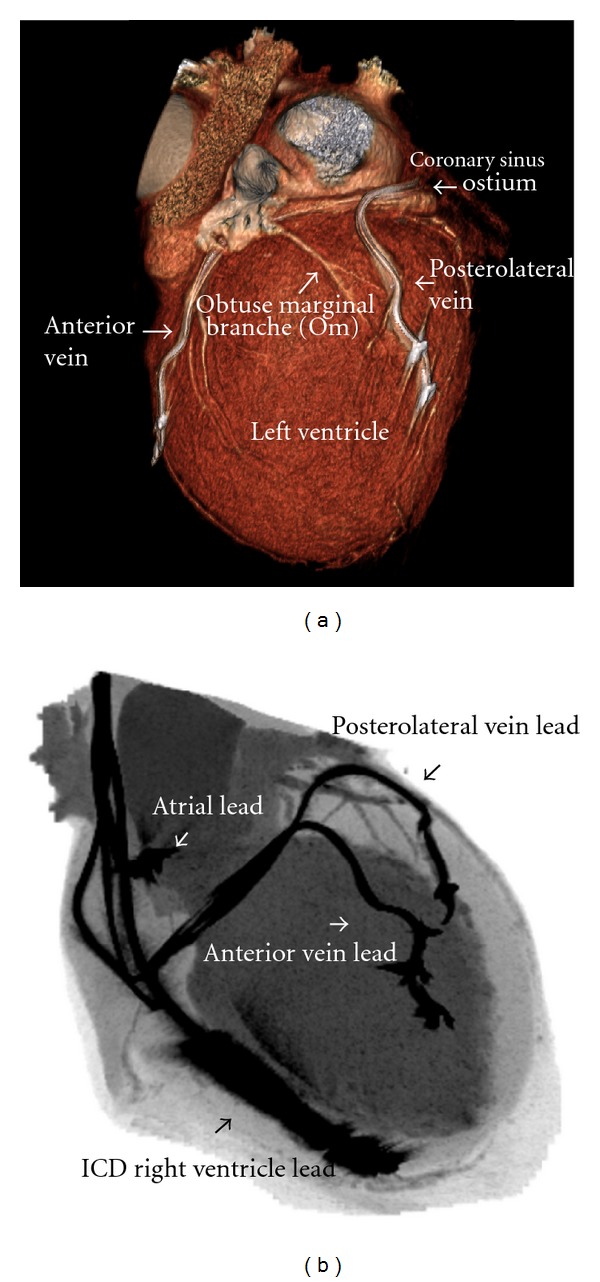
Computed tomography leads visualization: (a) 3D lateral view of the heart (volume rendering). Both LV leads are clearly visible. (b) Own reconstruction similar for intraoperational fluoroscopy. All leads visible.
